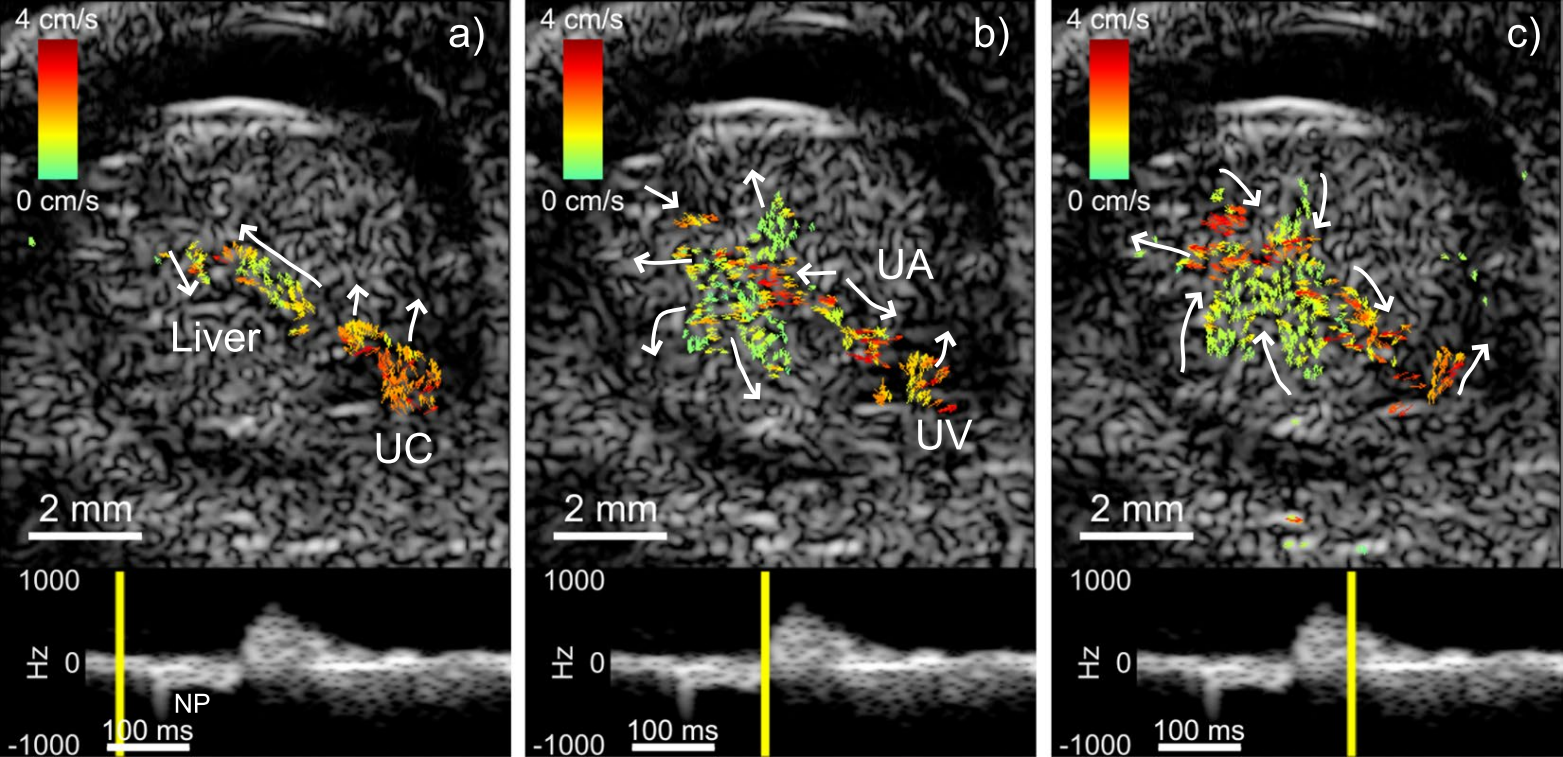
Three frames (40, 186 and 258 ms relative to start of spectrogram) from the vector flow analysis of the data sequence of Fig. 3. The Doppler spectrogram represents the same region of the liver as was indicated in Fig. 3a. The yellow line in the spectrogram indicates the slow-time point for which the data is displayed. (a) Diastole where the perfusion in the liver was minimal. (b) Late diastolic phase with a reversal of flow. (c) Systolic phase after the spectrogram peaked in the positive flow direction. The white arrows highlight the general direction of local flow vectors in each frame. Flow vectors point in the direction of flow and their color and length scale with the vector magnitude. NP: Negative pulse 93 ms after start of spectrogram, UA: Umbilical artery, UV: Umbilical vein. The full image sequence is available as Movie 2.
